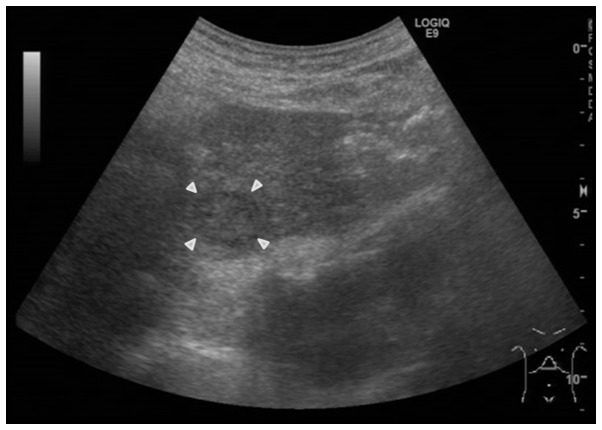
Abdominal ultrasound performed at the time of admission. A slightly poorly-defined high-echoic nodule 30 mm in size that was internally heterogeneous and comprised a low-echoic border was observed (arrowheads).
